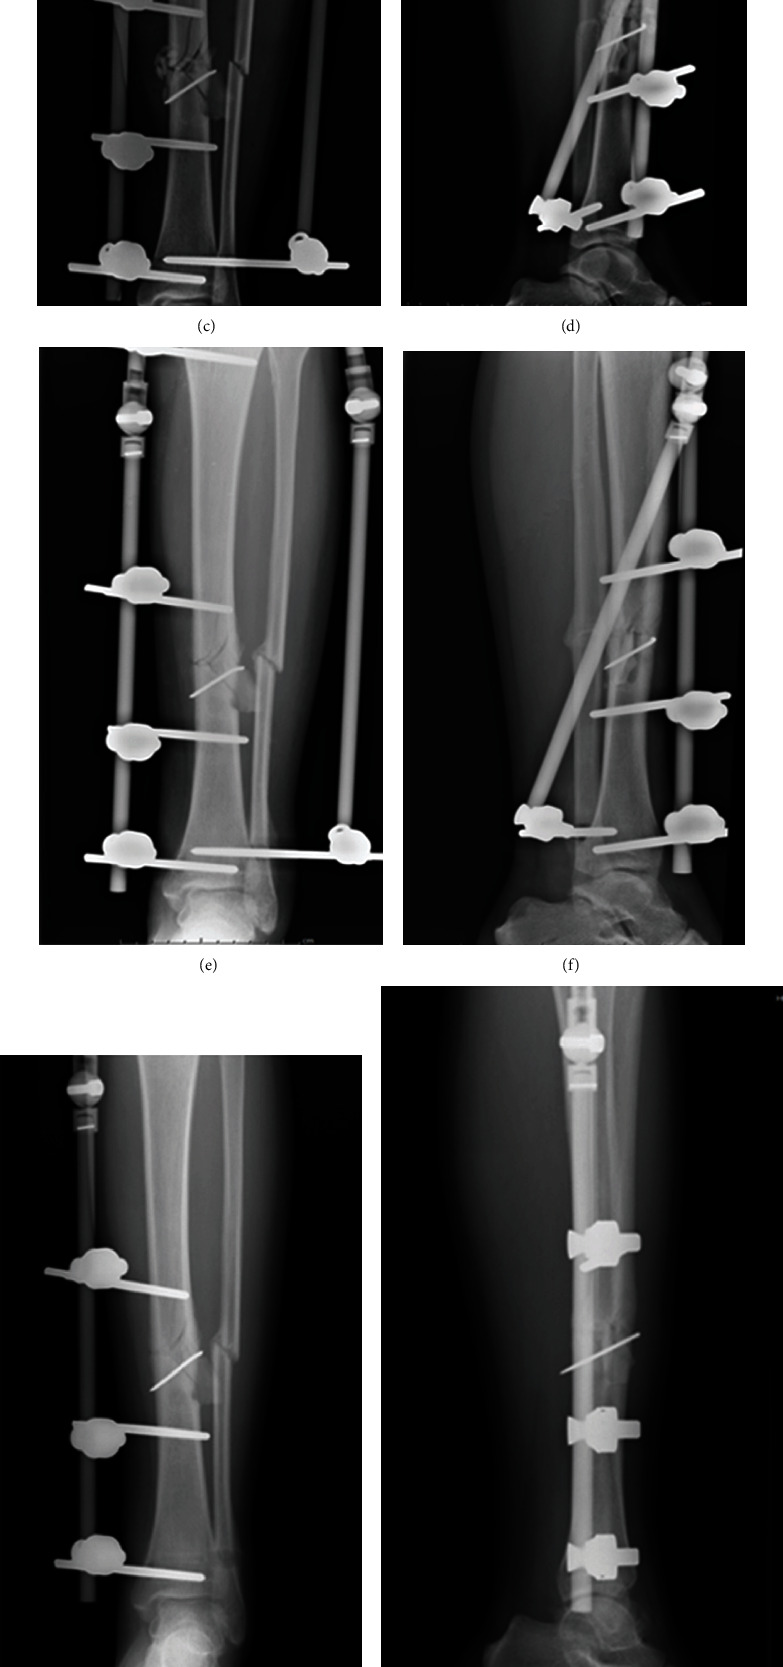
Representative images of good recovery after operation using unilateral external fixator combined with lateral auxiliary frame. The X-ray images at preoperation (a, b), after operation (c, d), postoperative 3 months (e, f), postoperative 6 months (g, h), and 12 months (i, j), respectively.
